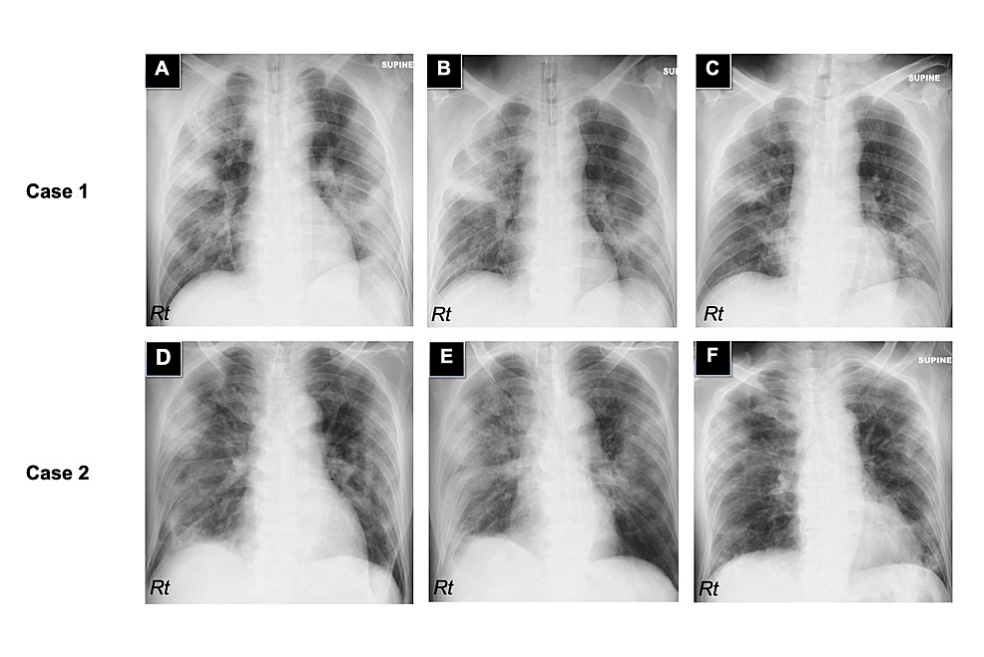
Imaging of methicillin-susceptible Staphylococcus aureus pneumonia in two COVID-19 patients. Chest X-rays of the first case on the fourth day of hospitalization (A), on the seventh day (B, before antibiotic therapy), and on the 16th day (C, after antibiotic therapy). Chest X-rays of the second case on the third day of hospitalization (D), on the sixth day (E, before antibiotic therapy), and on the 16th day (F, after antibiotic therapy). The infiltration shadows dramatically improved after antimicrobial treatment in both cases.
